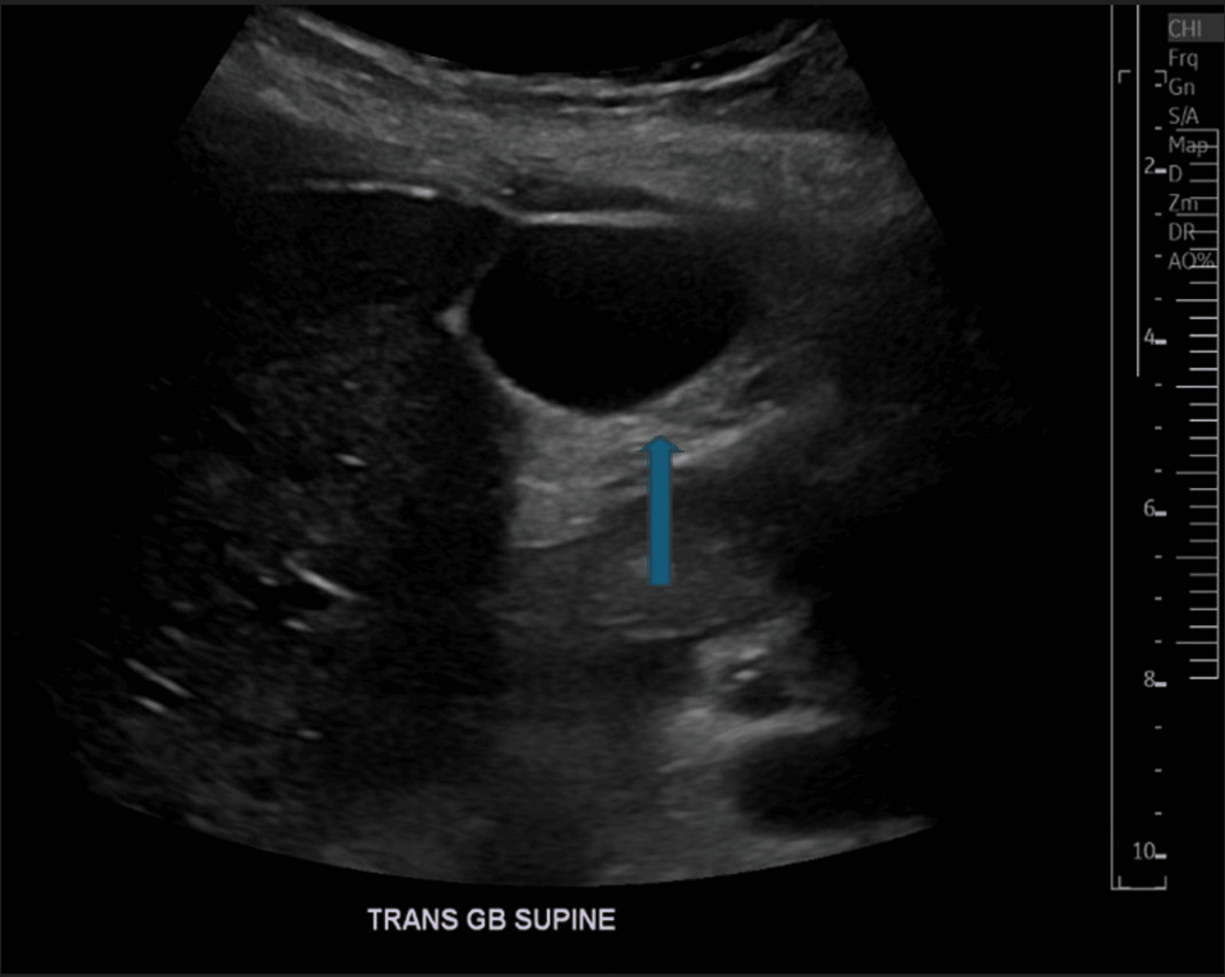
Ultrasound abdomen showing normal gallbladder with no gallstones (blue arrow).
